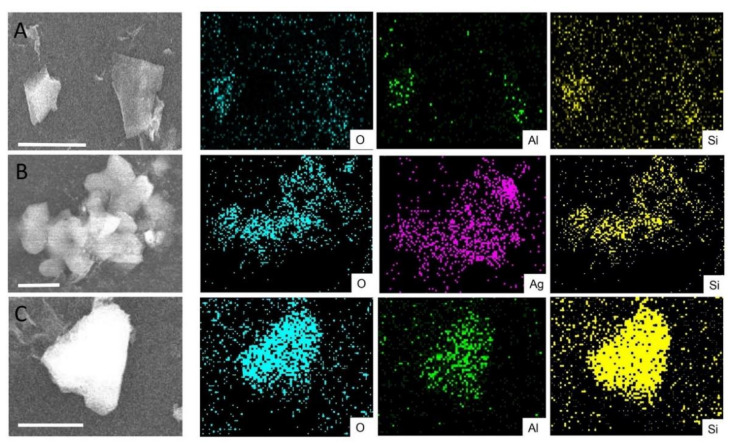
Energy-dispersive X-ray (EDX) mapping analysis of MMT (A), Ag_MMT (B) and Al_MMT (C). The reported scale bars correspond to 2.5 µm.
